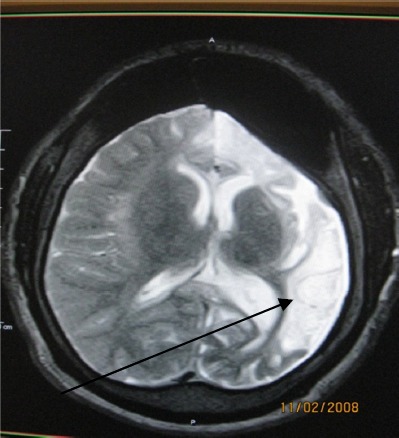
T2W axial MR image delineating a hypointense thin wall of a hyperintense left parietal lesion with surrounding hyperintense infarction
